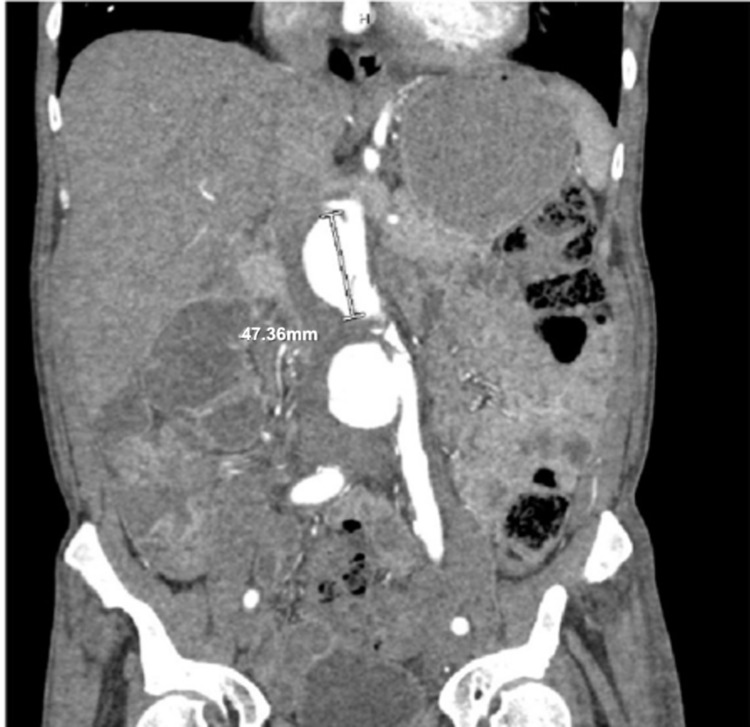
CT angiography abdomen demonstrating multiple infrarenal abdominal aortic aneurysms
